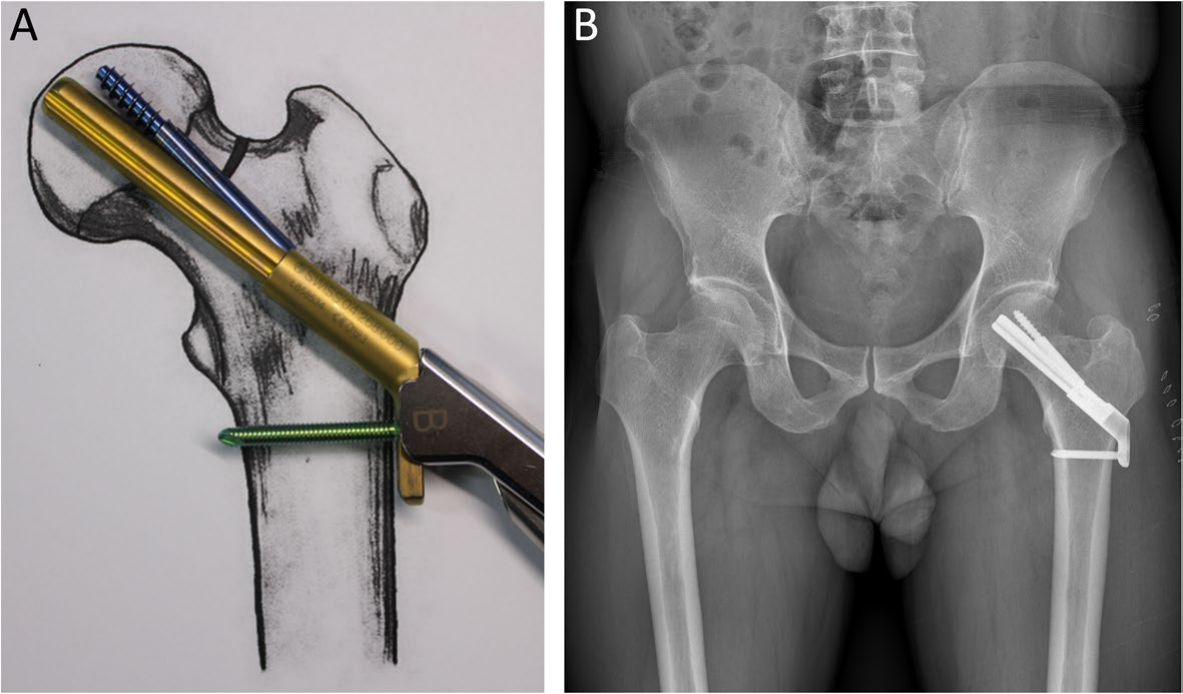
A Insert antirotation screw. B An immediate postoperative radiograph of in a 54-year-old male patient with femoral neck fracture who was treated with the Femoral neck system. The photographs were taken by the authors
